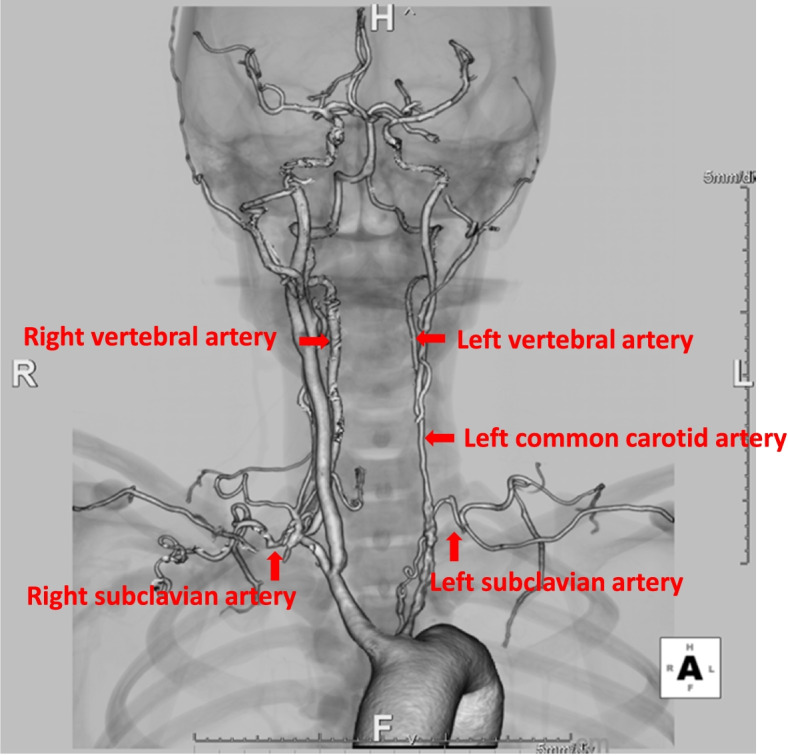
Three-dimensional computed tomography. Stenosis of the left common carotid artery, bilateral vertebral arteries, and bilateral subclavian arteries is shown (directional marker)
